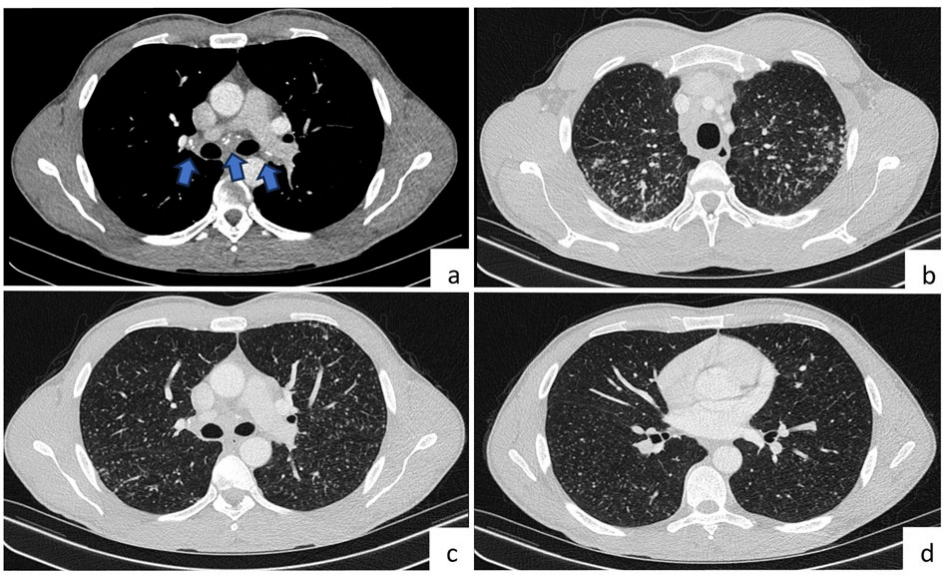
Pneumoconiosis. A 32-year-old male patient, who has no known disease and works as a dental technician, had incidentally detected lung CT findings compatible with silicosis. Axial lung CT images show calcified lymph nodes in the mediastinum and bilateral hilar regions (a, arrows), and multiple millimetric solid nodules predominantly in the upper lobes and periphery of both lungs (b–d)
